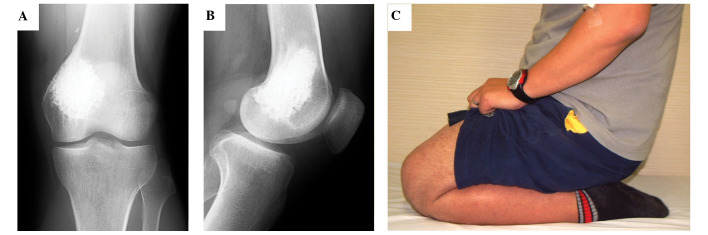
Repeated (A) anteroposterior and (B) lateral radiographs of the distal femur demonstrate no recurrence after the 12-year follow-up period. The radiolucent area between the implanted hydroxyapatite and the surrounding cancellous bone is completely absent. (C) Clinical range of motion of the knee; the patient is able to sit back on his heels with a straight back without experiencing pain.
